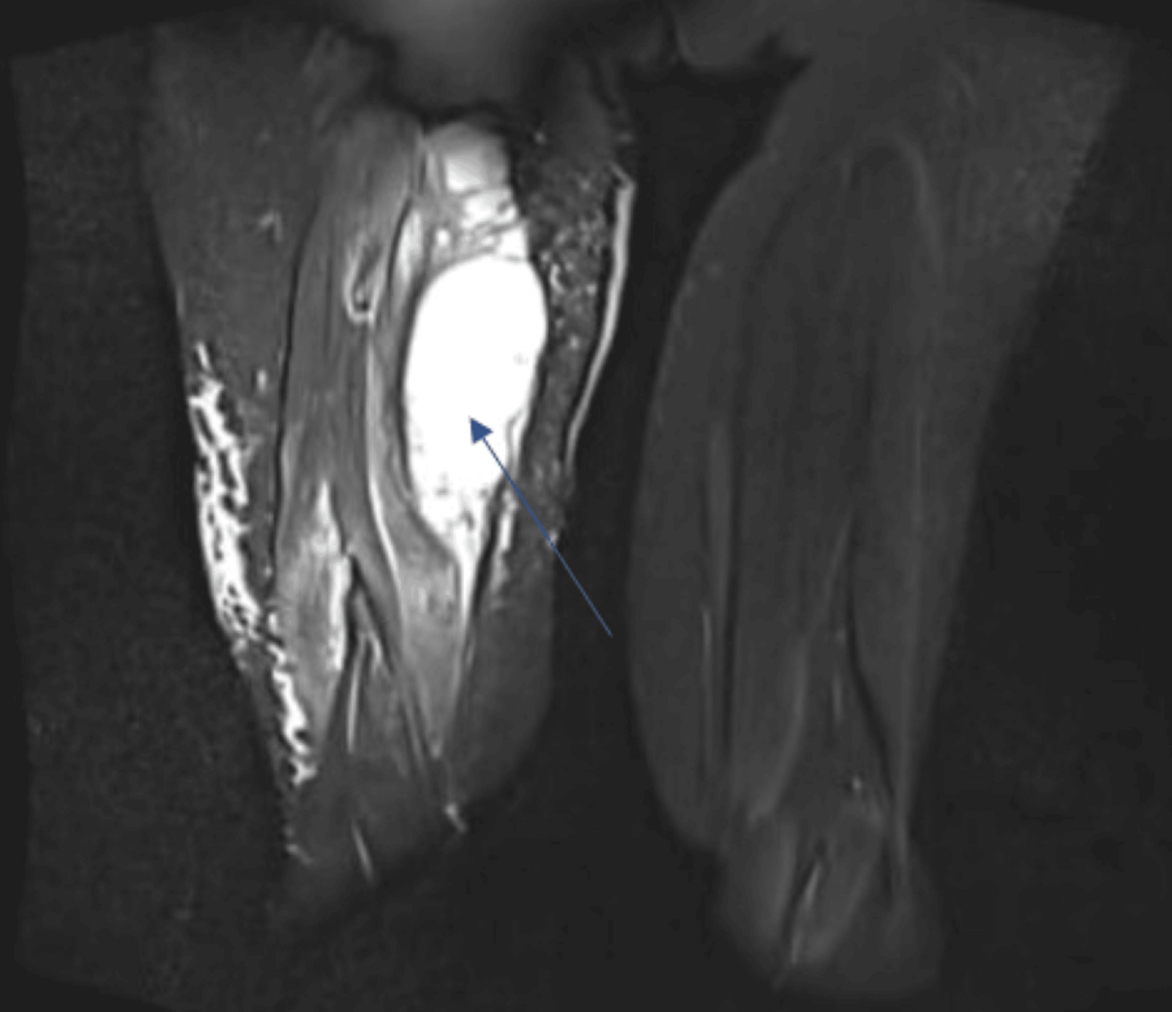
MRI of the right thigh in coronal section (stir sequence) showing hyperintensity (blue arrow)
